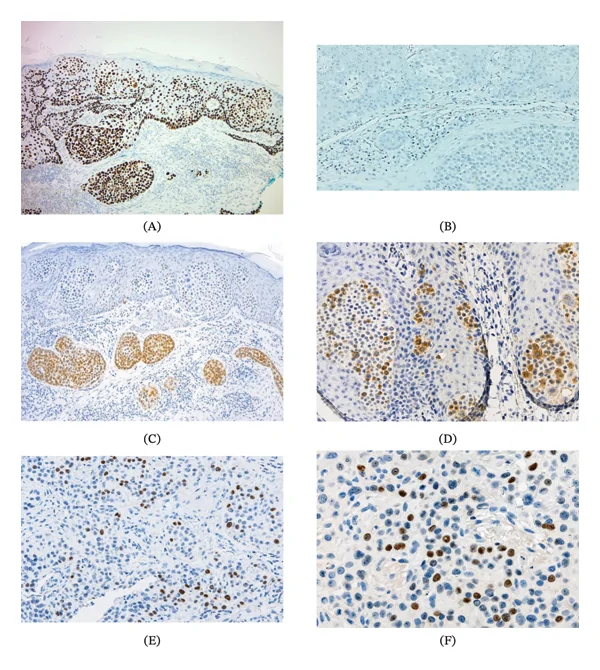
(A) P63 nuclear positivity of the neoplastic cells in the dermis and epidermis. 100x. (B) Negative reaction for PSA in the neoplastic cells. 100x. (C, D) PSMA cytoplasmic and membranous positivity 100x and 400x, respectively. (E, F) NKX3.1 nuclear positivity 100x and 400x, respectively.
